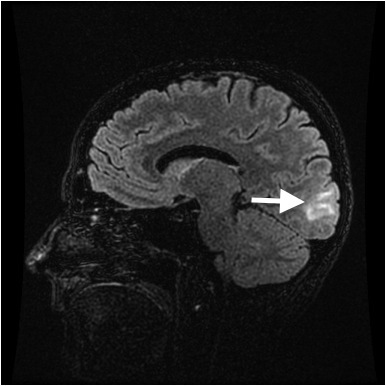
T2 weighted magnetic resonance imaging of Patient 1, showing hyperintensity within the occipital lobe.
